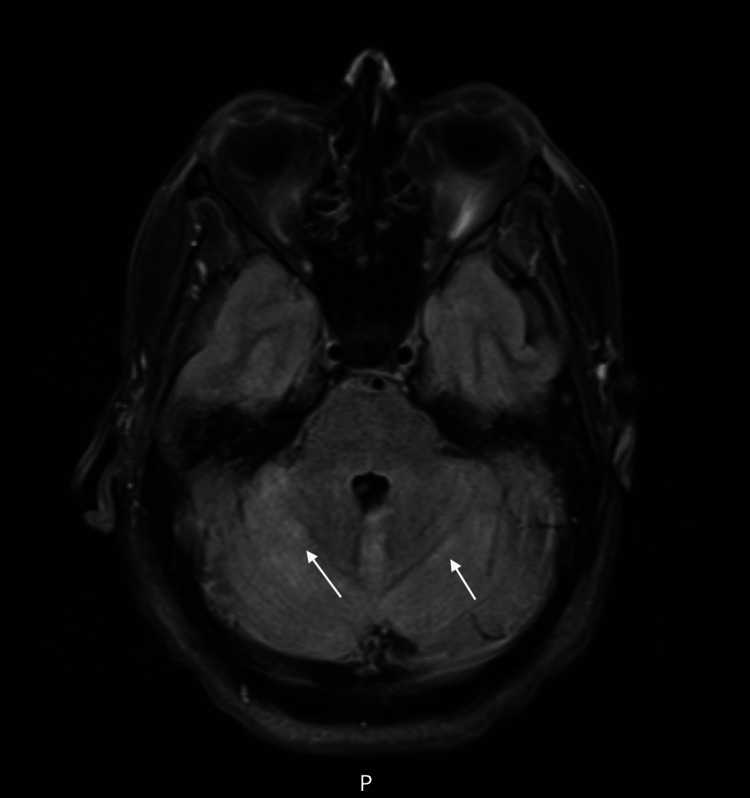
Hyperintense signals in the cerebellum (arrows) on FLAIR MRI of the brain, axial view FLAIR: Fluid-attenuated inversion recovery; MRI: magnetic resonance imaging
